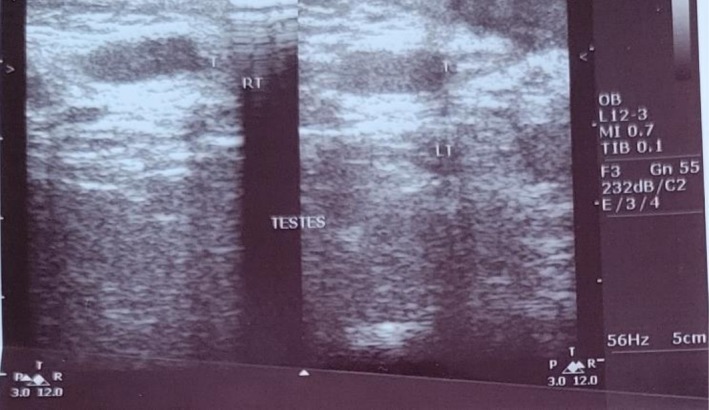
Follow‐up scrotal ultrasound showing both testes correctly positioned within the scrotum.
